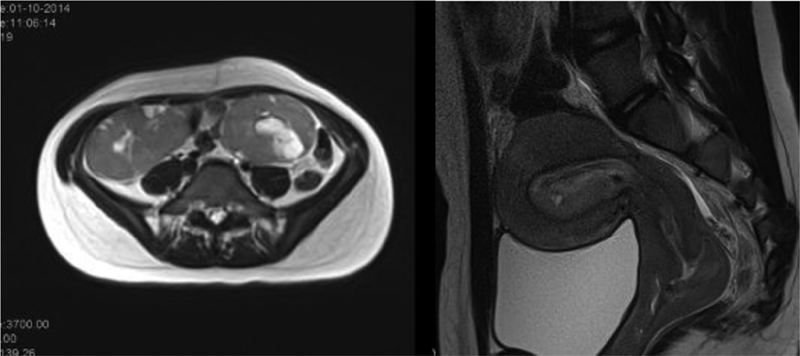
Computed tomography images with evidence of uterine and ovarian involvement due to previously diagnosed Burkitt lymphoma.
